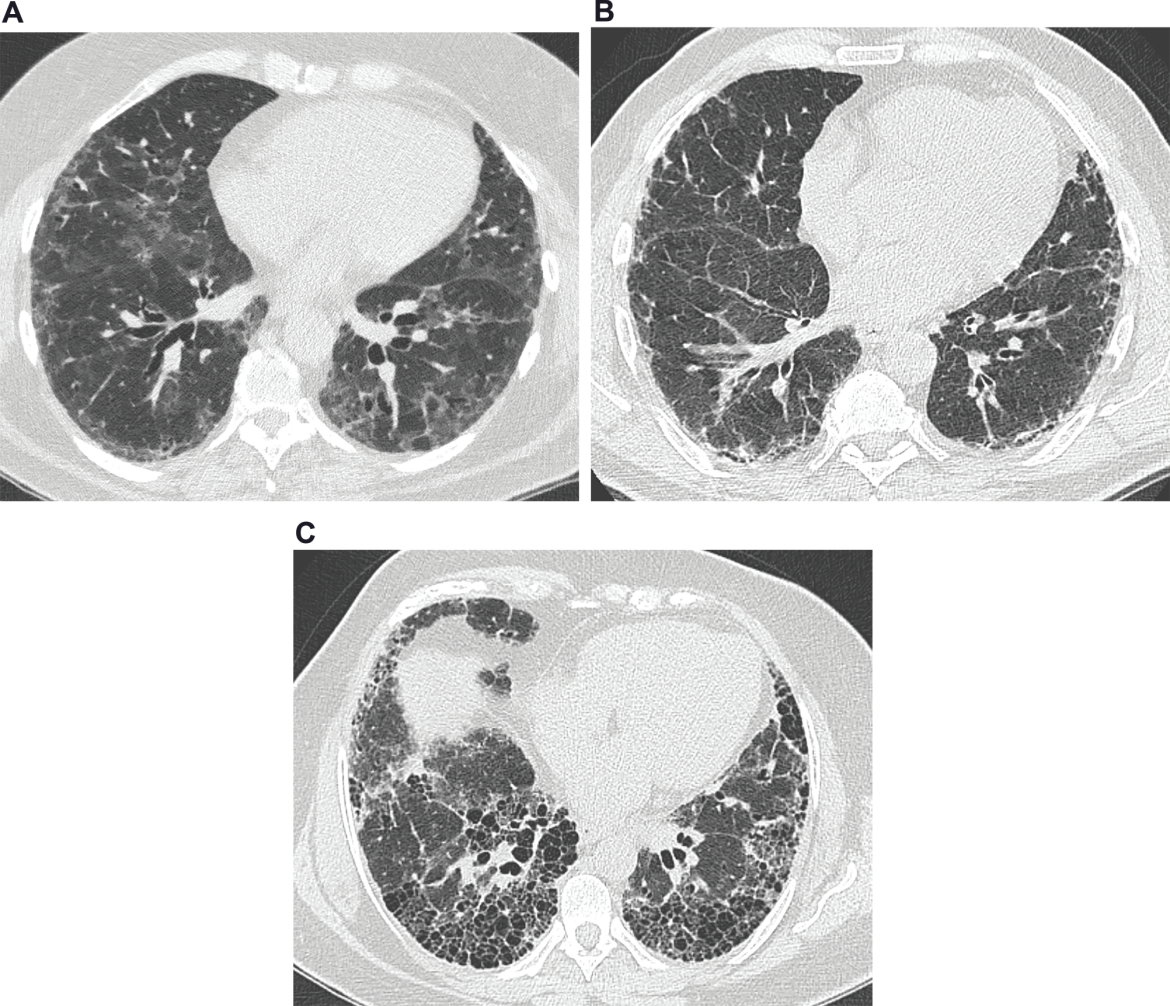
Common features on high-resolution computed tomography in interstitial lung diseases. (a) Images from a 63-year-old female presenting a nonspecific interstitial pneumonia pattern. There are predominant areas of ground-glass opacities, with some traction bronchiectasis and cortical interlobular septal thickening. (b) Images from a 61-year-old male with idiopathic pulmonary fibrosis. There are diffuse areas of interlobular septal thickening, predominantly in the cortical lung zones. (c) Images from a 56-year-old female with idiopathic pulmonary fibrosis. There are extensive areas of honeycombing, with some interlobular septal thickening.
